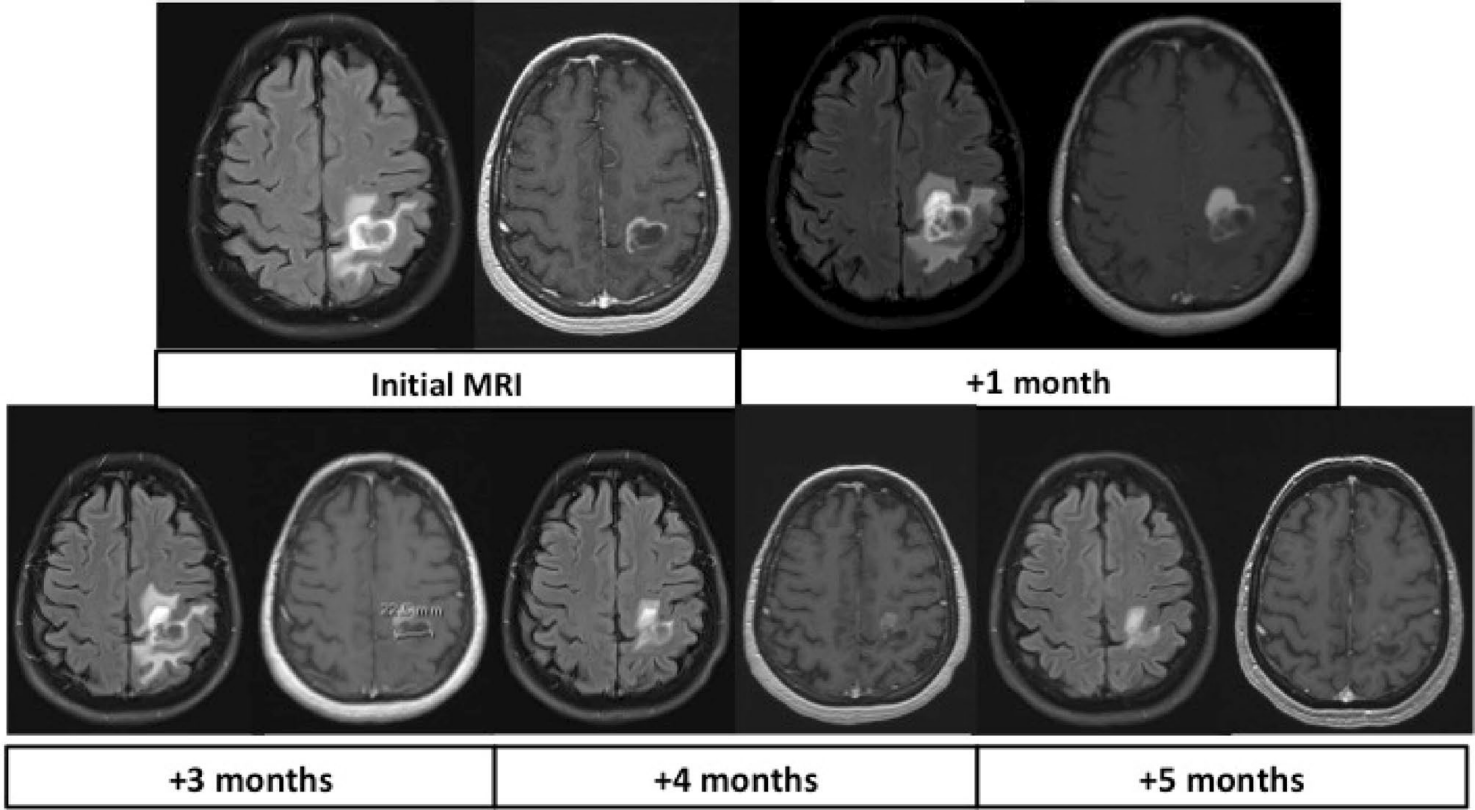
Patient 1 (N1) brain MRI (FLAIR on the left and T1 gadolinium on the right) showing progressive reduction of pseudotumoral lesion size and contrast enhancement over time, while receiving rituximab infusions
